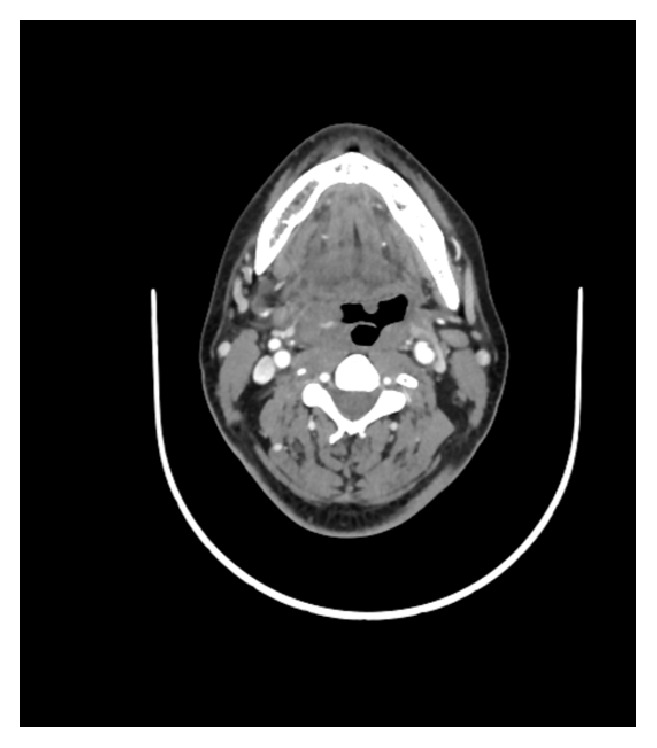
Axial cut of contrast enhanced CT scan of paranasal sinuses showing involvement of parapharyngeal space by Juvenile Nasopharyngeal Angiofibroma.
